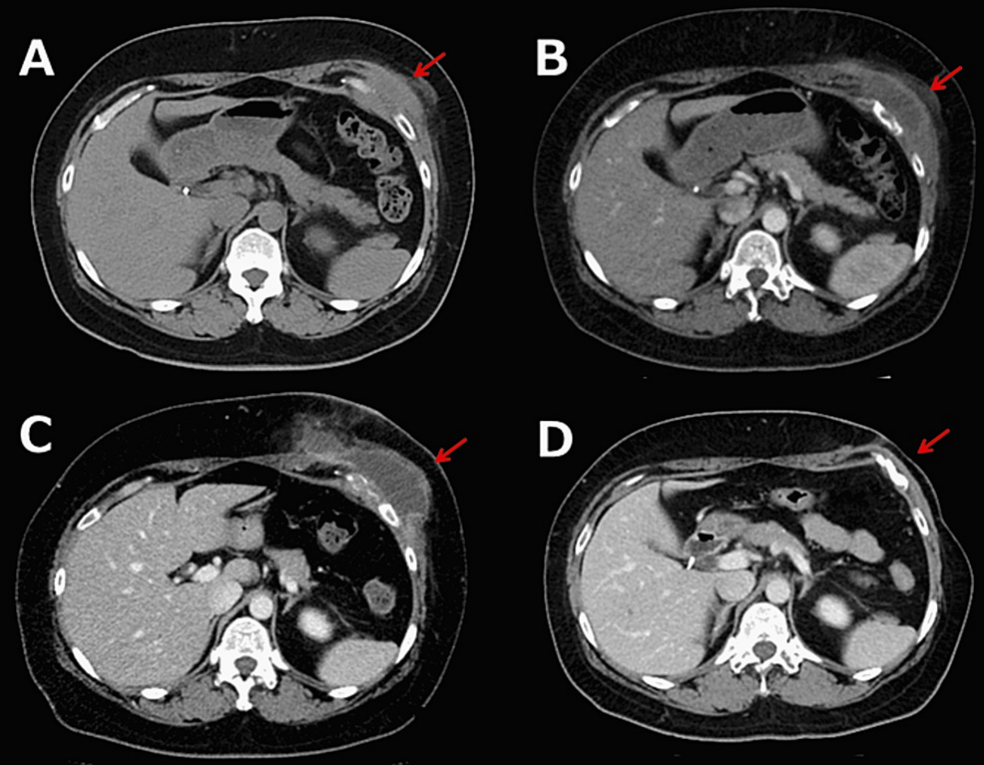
Whole-body computed tomography (CT) shows the course of the left subcutaneous abscess. (A) A whole-body CT at the previous hospital where the Lap-C was performed. It revealed a fluid density area around the lower left rib in the anterior chest wall, which was suspected to be a “hematoma”. (B) A contrast-enhanced whole-body CT on admission revealed a fluid density area around the lower left side of the ribs. (C) The subcutaneous abscess grew again 20 days after discharge. (D) The subcutaneous had completely resolved. Red solid arrows indicated the subcutaneous abscess.
